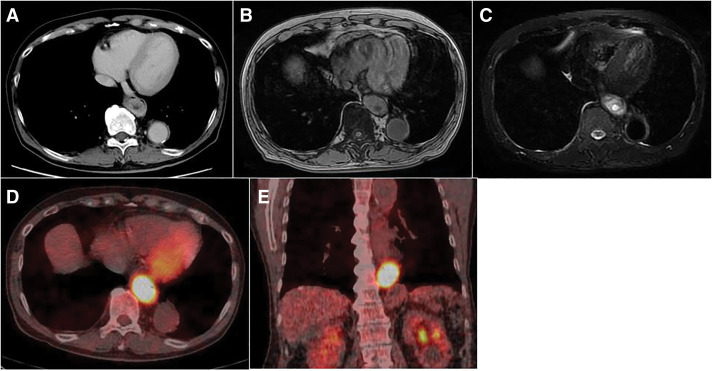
(A) Contrast-enhanced CT scan revealed a 60-mm SOL protruding into the lumen of the lower esophagus. The SOL exhibited peripheral enhancement with a central low-density area. (B, C) MRI revealed a SOL in the lower esophagus with high signal intensity on T2-weighted images and moderate signal intensity on T1-weighted images. The lesion contained cystic components exhibiting high T2 and low T1 signal intensities. (D, E) FDG-PET/CT revealed intense FDG uptake in the early phase, which further increased in the delayed phase (SUVmax: 11 → 18). SOL, space-occupying lesion; SUVmax, maximum standardized uptake value
